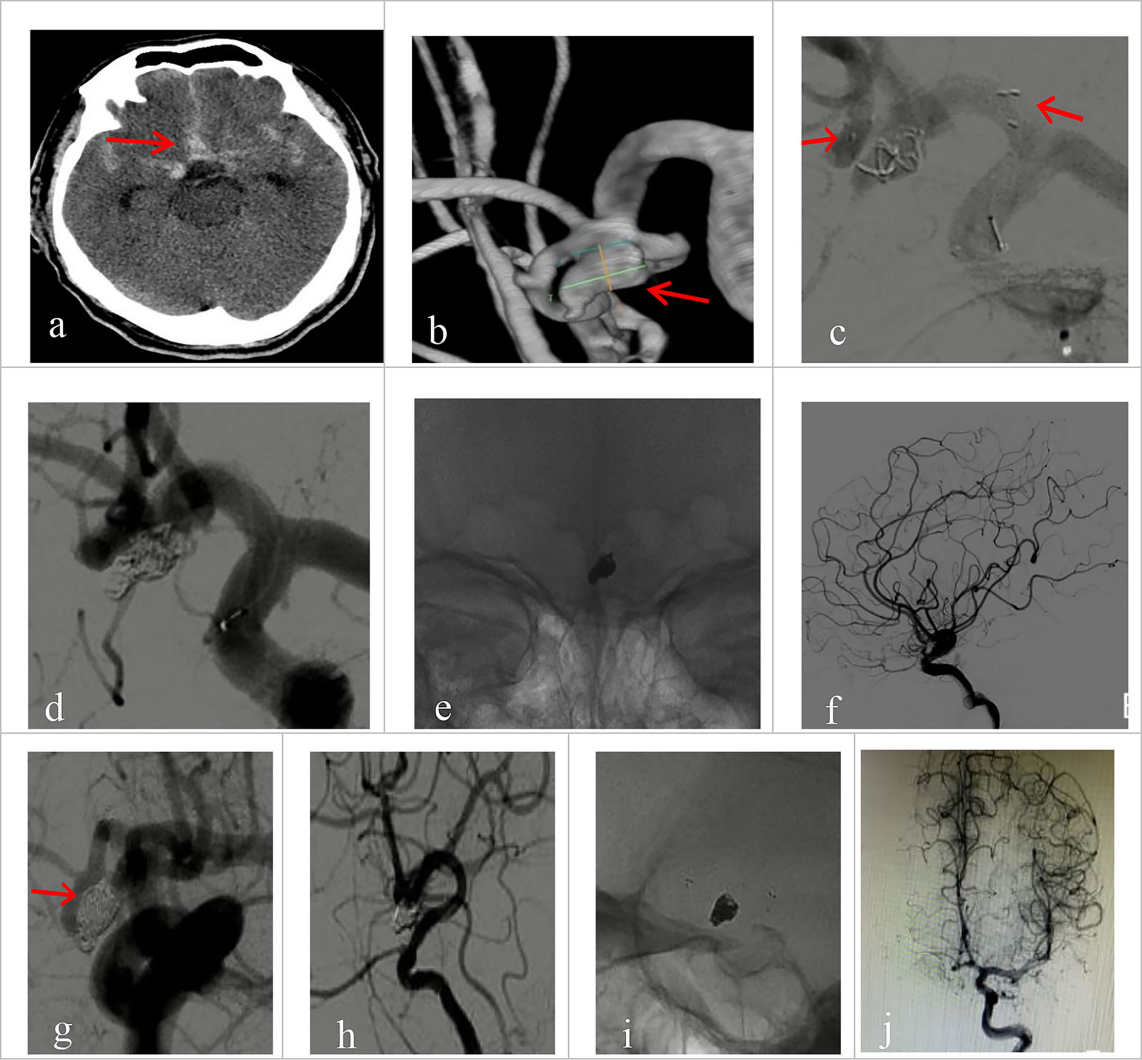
Imaging data of patients with ruptured wide-necked anterior communicating artery (ACoA) aneurysm treated with Neuroform Atlas assisted coiling (dual catheter system). (a) Preprocedural head CT showed spontaneous subarachnoid hemorrhage (red arrowhead). (b) Preprocedural DSA angiography with 3D reconstruction showed the location of a wide-necked ACoA aneurysm (red arrowhead). (c) The stent was positioned as planned and was accurately deployed during the procedure. The left red arrowhead indicates the proximal end of the stent, and the right arrowhead indicates the distal end. (d) The coil was delivered after the stent was deployed. (e) The stent maintained its expansion after coiling. (f) Immediate post-operative angiography (lateral view) demonstrated good patency of the aneurysm-bearing artery and its branch vessels without deficits. (g–j) Immediate angiography control showed that the aneurysm was completely embolized (Raymond Grade I), with sound patency of the stent, free of thrombosis. Vessels at all grades had good filling. (g) No coil compression 6 month after the procedure was seen on angiography at working projection (red arrowhead indicates the neck of the aneurysm). (h) Satisfactory embolization of the aneurysm with no recurrence, no contrast agent stagnation, no thrombosis in the stent. (i) Good expansion of the stent on 3D rotating view, without coil compression. (j) Parent vessel and its branches had normal fill.
